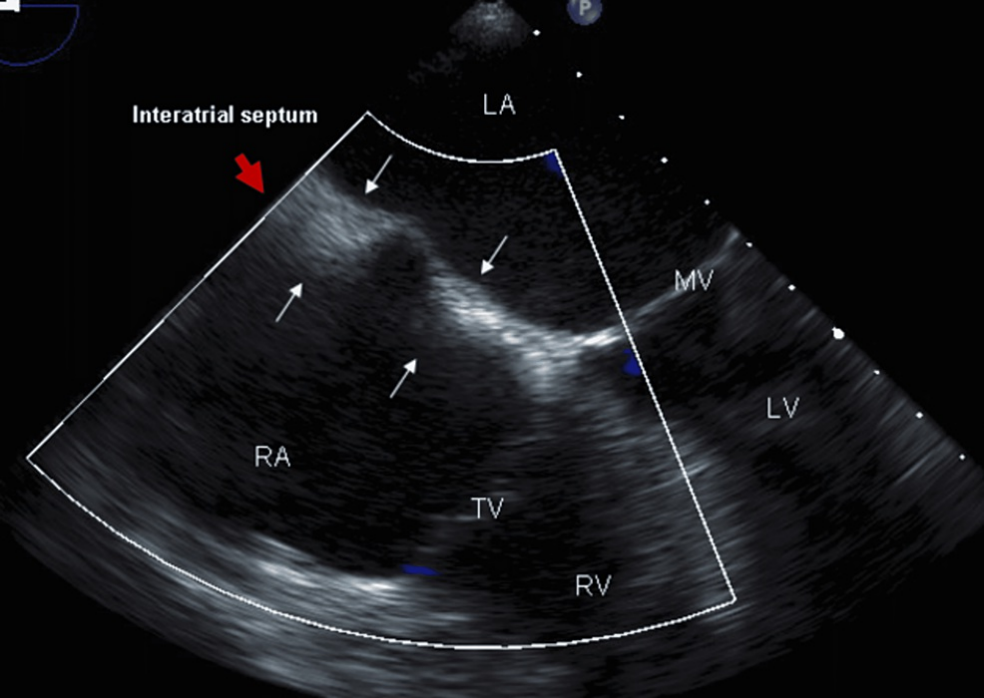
Transesophageal echocardiogram revealing thickened interatrial septum with sparing of fossa ovalis, consistent with lipomatous hypertrophy of interatrial septum. LA: left atrium; RA: left atrium; LV: left ventricle; RV: right ventricle; TV: tricuspid valve, MV: mitral valve
